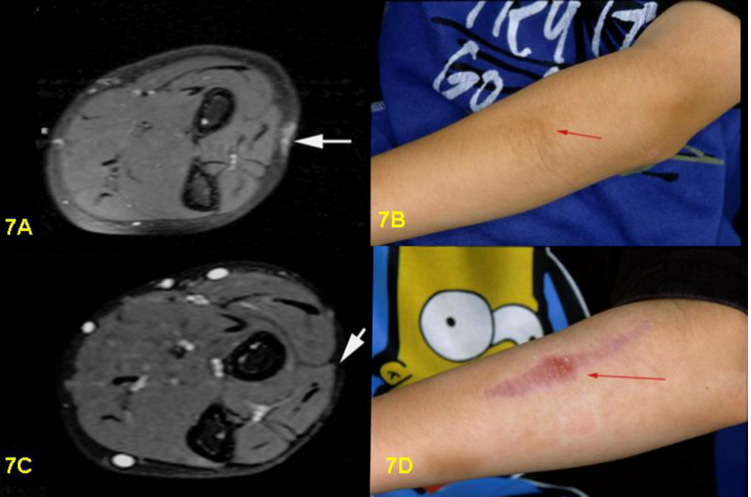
7A: Pre-surgical T2-weighted STIR-sequence, transversal section: Cutaneous and subcutaneous PNF of forearm, bright signal without involvement of muscles or fascia. 7B: Pre-surigcal clinical view of hyperpigmented PNF of left forearm. 7C: Post-surgical T2-weighted STIR-sequence, axial section: complete removal of tumor. 7D: Post-surgical clinical view of scar on left forearm.
